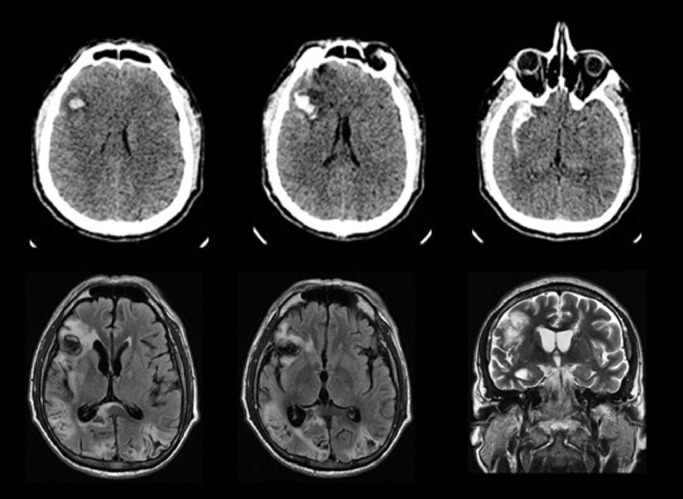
Image studies. Three CT axial sections show a heterogeneous area of ​​intraparenchymal and subarachnoid bleeding, and intracerebral hematoma in the right frontal lobe. Two axial MRI sections in a FLAIR sequence show the extension of the lesion, involving the lateral aspects of the right frontal lobe, with extension to deep white matter, the anterior insular cortex, and the right occipito-temporo-parietal cortex, as well as hemorrhagic infarction in both occipital lobes. A coronal T2 MRI section shows the lesion in the lateral aspect of the right hemisphere
